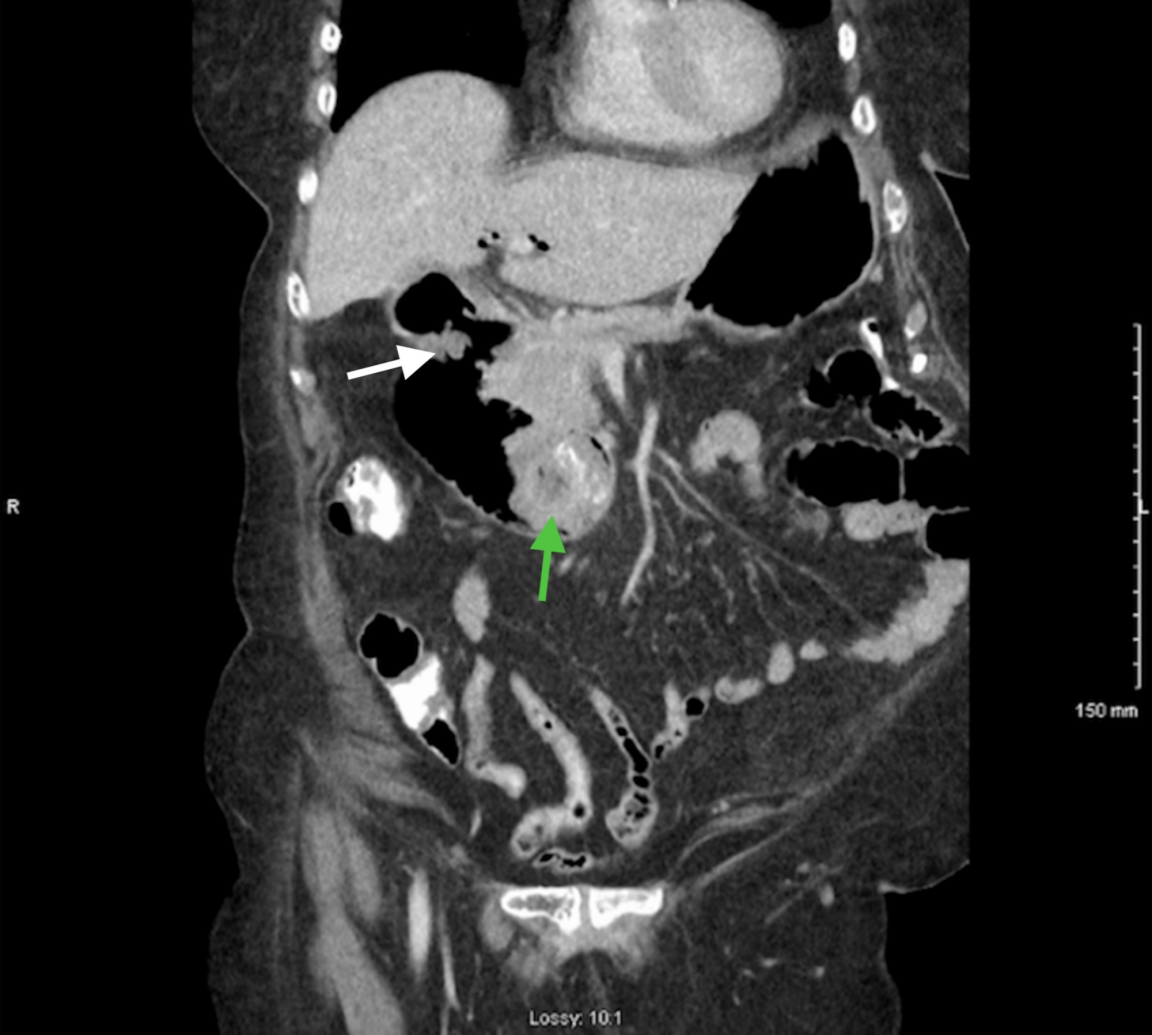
Follow-up computed tomography scan three years after initial presentation showing complete erosion of the stone into the duodenum with gastric distention (white arrow indicating the fistula and green arrow indicating the stone)
